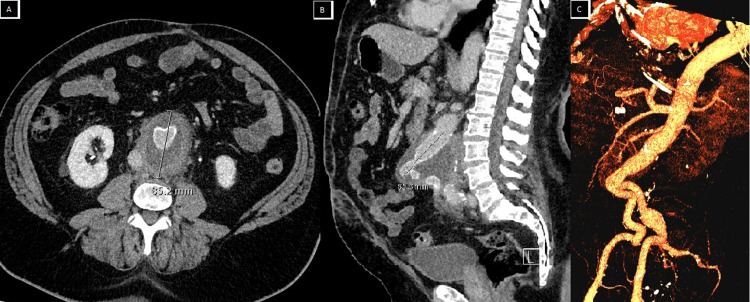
CT-angiogram following open repair in axial (A), sagittal (B), and reconstruction (left oblique) (C) views.
